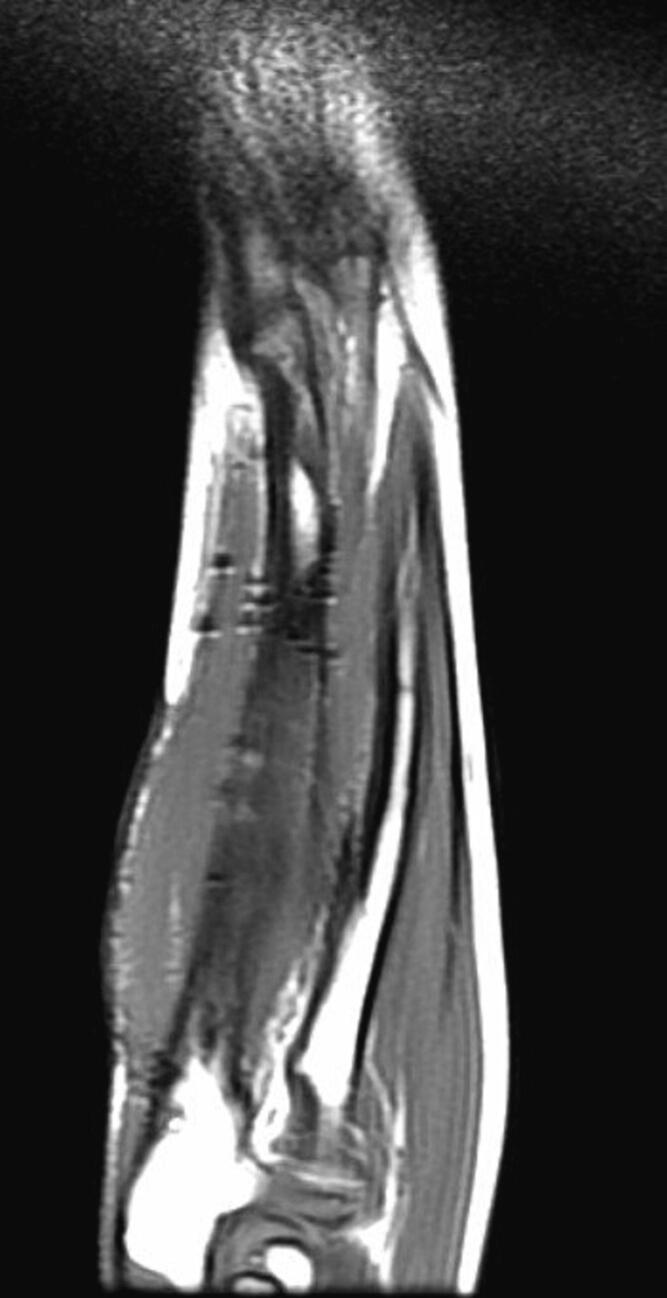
Postoperative MRI indicates the total removal of the FCD (frontal view).
